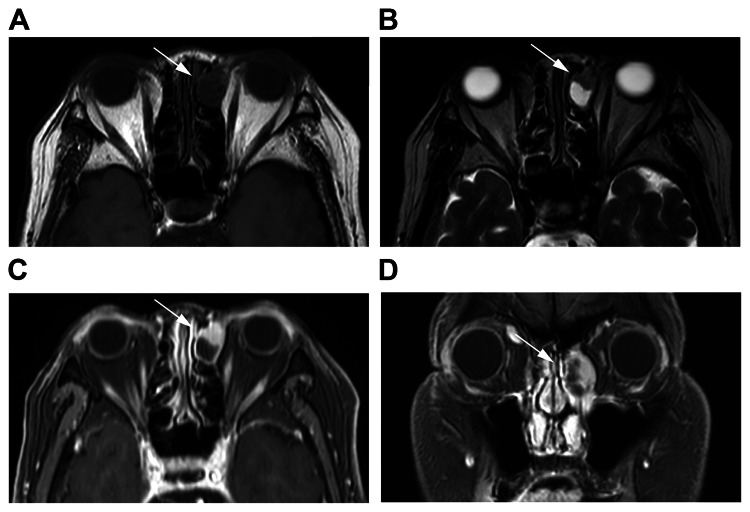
MRI scan of the paranasal sinuses: (A, B) axial T1-weighted images and (C, D) coronal T2-weighted images A lesion with a nearly isointense signal compared to muscle on T1-weighted images and a mixture of hypo- and hyperintense regions on T2-weighted images is seen. MRI: magnetic resonance imaging Source: Image of the patient showing the condition/feature. Informed consent for the use of this image was obtained from the patient.
